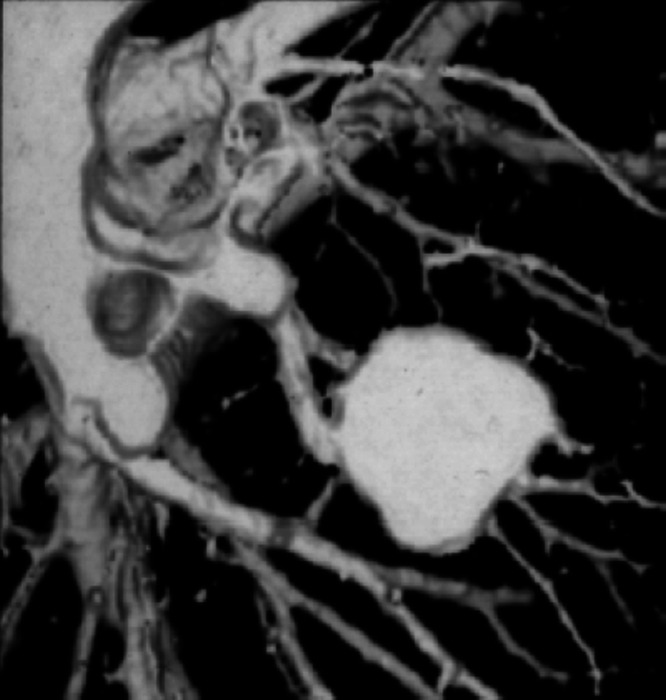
First spiral CT scan of the lung presented at RSNA 1989 (courtesy of Willi Kalender).
